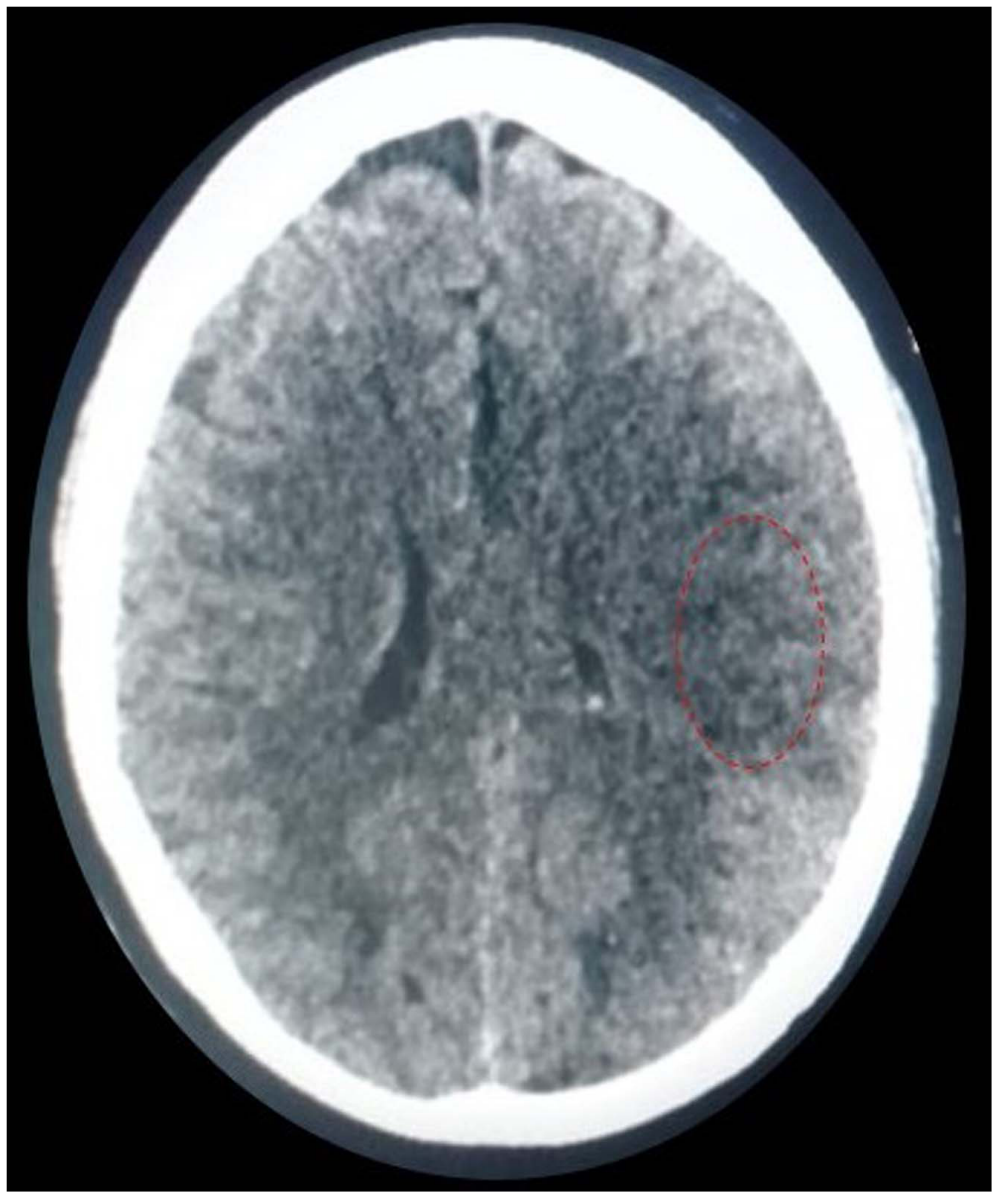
Post-thrombectomy; non-contrast CT scan showing subtle hypodensity in left MCA territory with an ASPECT of 9.
